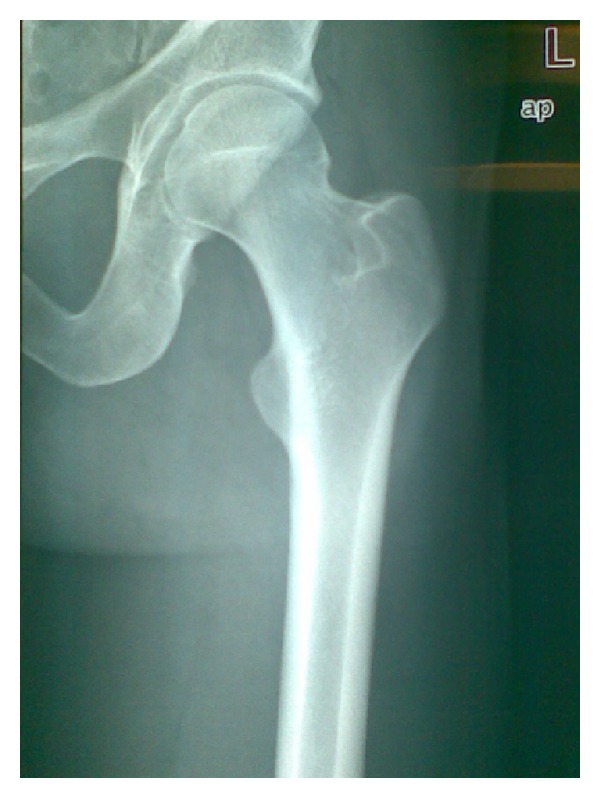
Plain X-ray of the thigh showing no significant soft tissue abnormalities.
